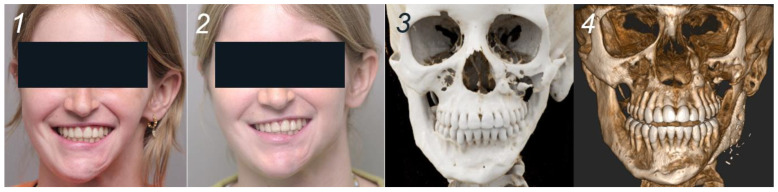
Clinical and radiological (CBCT) situation preoperatively and after 2.5 months, case 2. (1): Clinical situation pre-surgery—frontal plane. (2): Clinical situation 2.5 months post-surgery. (3): Radiological situation pre-surgery. (4): Radiological situation 2.5 months post-surgery.
